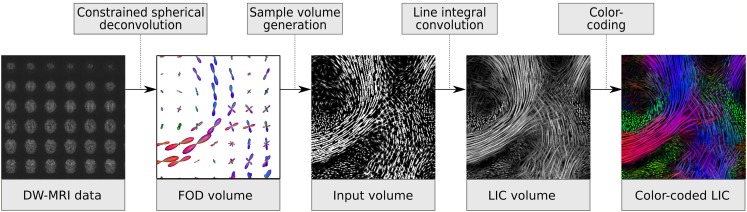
Processing steps of the A-Glyph LIC method. From DW-MRI data, the FOD volume is calculated by constrained spherical deconvolution. Then, an anisotropic glyph sample volume is generated and used as input pattern for multiple kernel line integral convolution. The LIC result is then color coded
